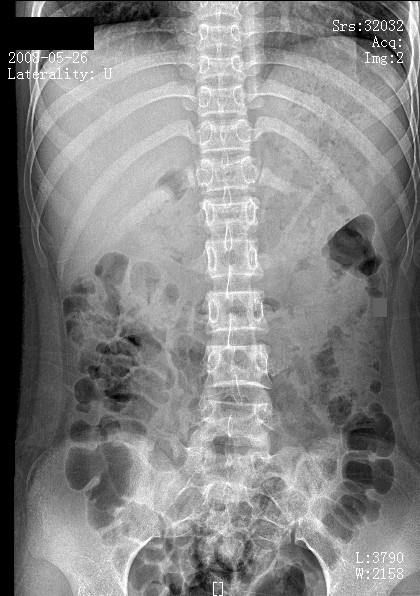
Pre-operative radiograph (anteroposterior view) with thoracolumbar vertebral collapse.
